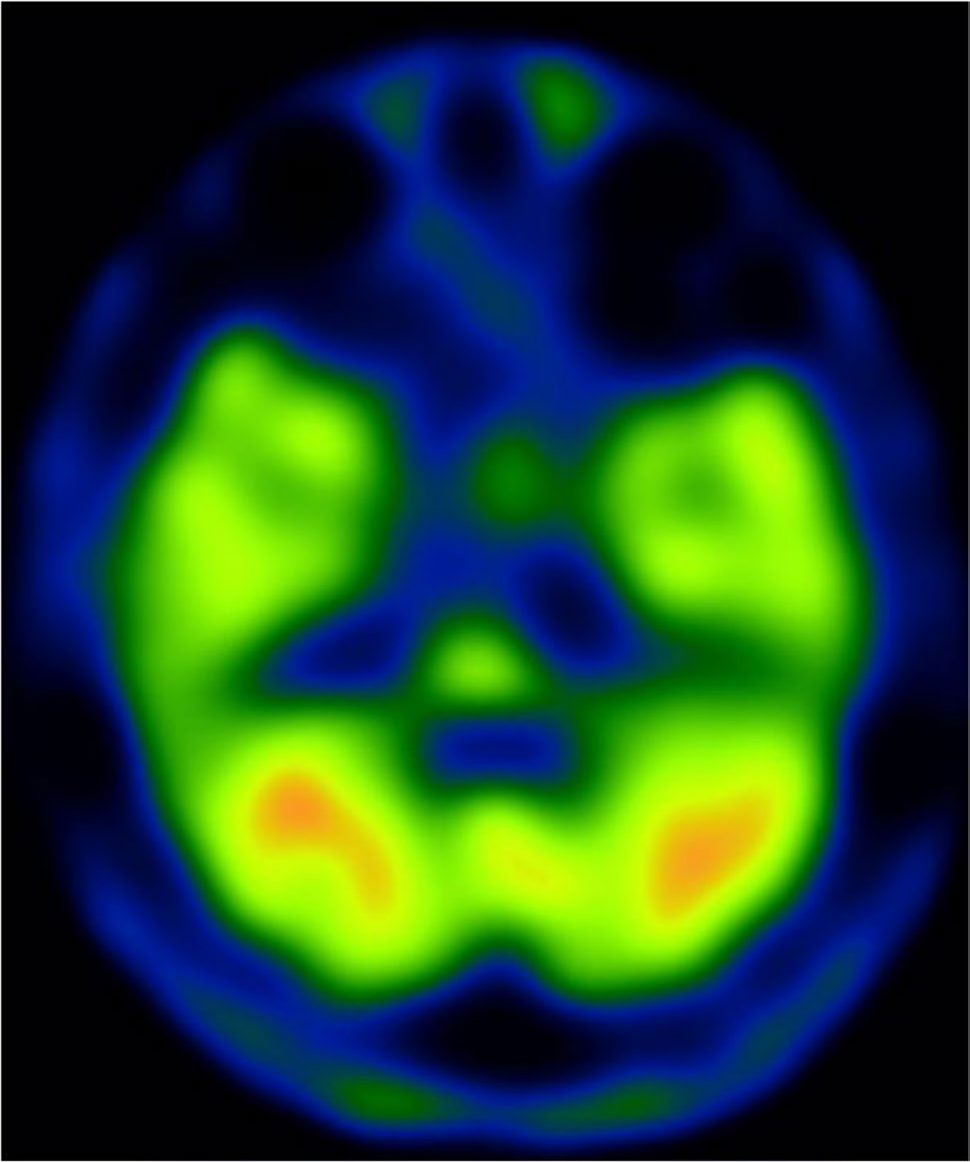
Brain single-photon emission computed tomography (SPECT). Brain SPECT shows decreased cerebral blood flow in the cerebellum, vermis, and brainstem, which are associated with SCA3
